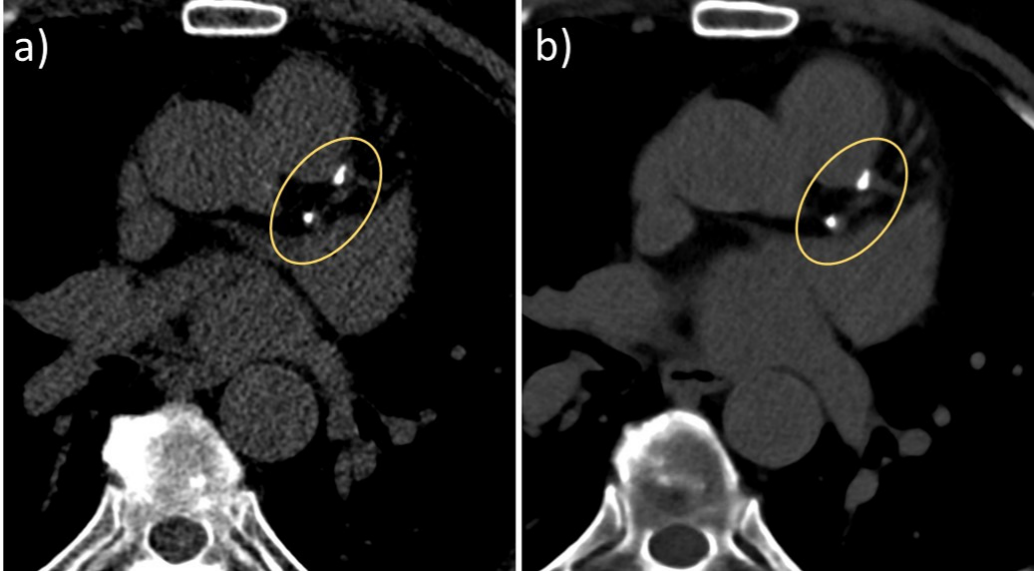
Clinical example of CAC scoring with PCD-CT. (a) Non-contrast CAC scoring scan of a 73-year-old male patient (120 kVp, 70 keV, 3 mm slice, Qr36, 61 bpm). Agatston score 322. (b) VNI image from a contrast-enhanced scan (120 kVp, 70 keV, 3 mm slice, Qr36, 57 bpm). Agatston score 330. Note the reduced image noise in the VNI image because of the higher radiation dose of the contrast-enhanced scan. CAC, coronary artery calcium; PCD-CT, photon-counting detector CT; VNI, virtual non-iodine.
